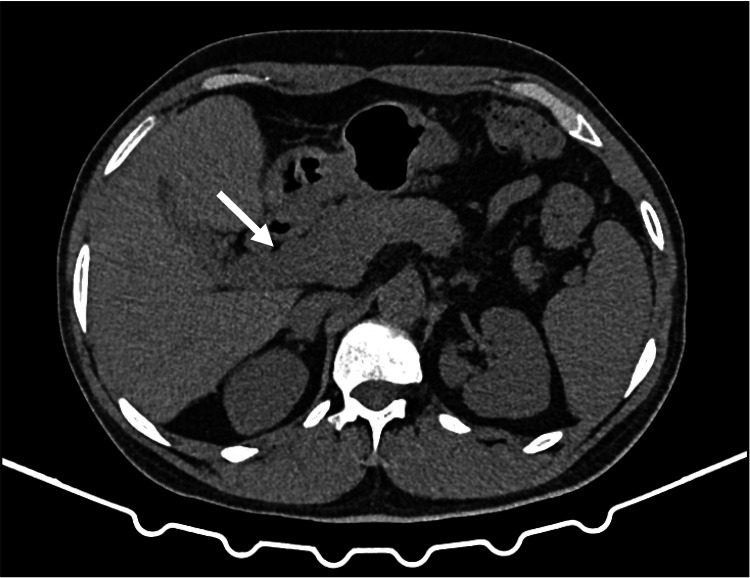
Follow-up abdominal CT (axial view) Axial CT image obtained on follow-up demonstrates improvement of the previously described pancreatic inflammatory changes (arrow) CT: computed tomography
